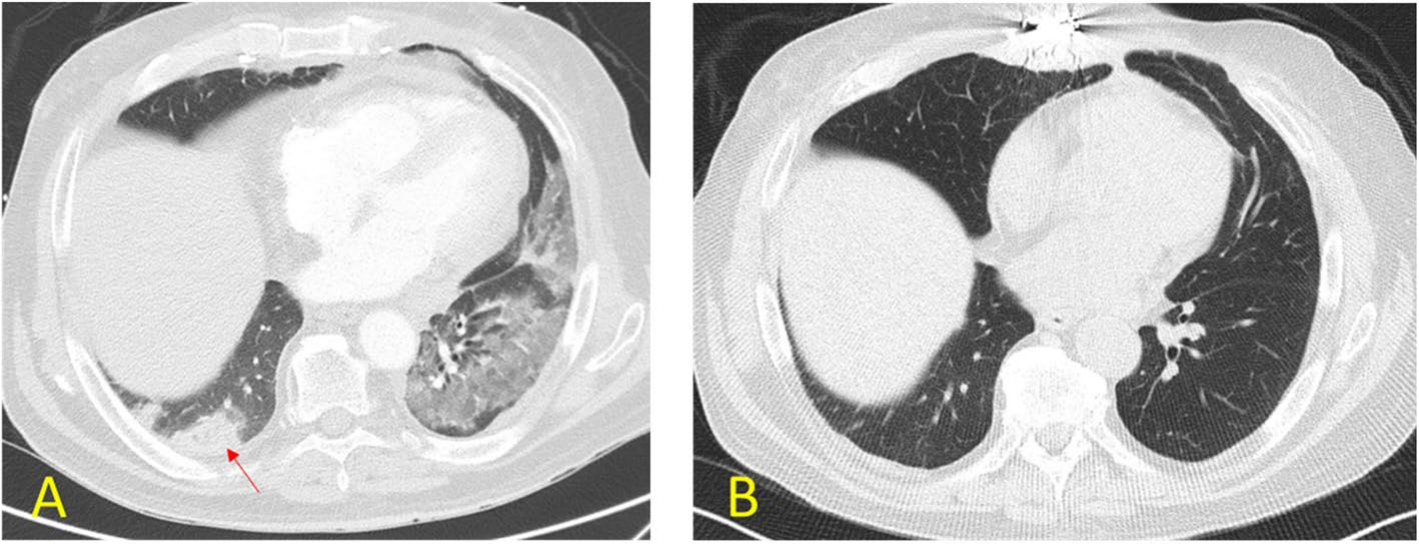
CT scan of the chest before and after thrombectomy in a case of acute PE in a lung transplant patient. 2A: CT scan before thrombectomy showing new ground glass and consolidation involving the lower lobes suggestive of ischemia with development of infarction (Arrow). 2B: CT scan 2 months after thrombectomy showing interval resolution of previously seen infiltrate/consolidation of the lower lobes
